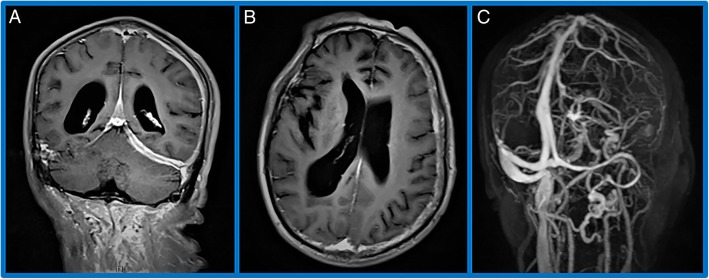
Magnetic resonance imaging of the brain: a thickening of dura mater localized at the left tentorium cerebelli, left cerebral hemisphere, and cerebral falx; the thickening dura mater was characterized by an intense contrast enhancement after the administration of gadolinium (a, b). Magnetic resonance venography: no obvious indication of the left internal jugular vein, and both of the left transverse sinus and the sigmoid sinus was slim with local stenosis; there was also increased cortical drainage in the left cerebral hemisphere (c)
